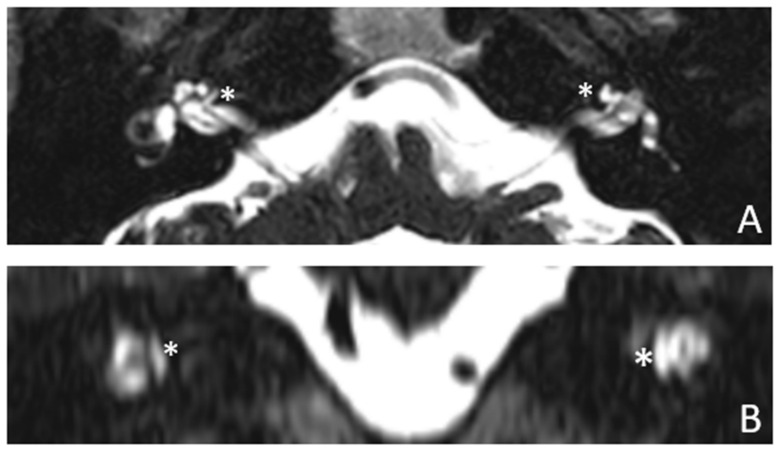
MR images of patient #2 with osteogenesis imperfecta. MR cisternography: (A), axial plane; (B), coronal plane. Note the fluid-signal in the newly formed cavities (white asterisk).
